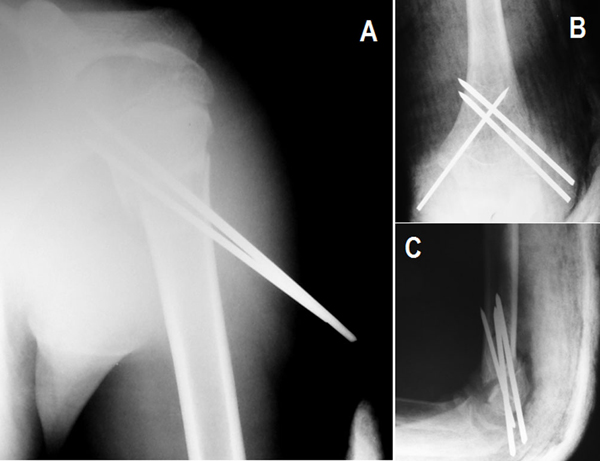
Early postoperative roentgenograms of (A) left shoulder and (B, C) left elbow.
